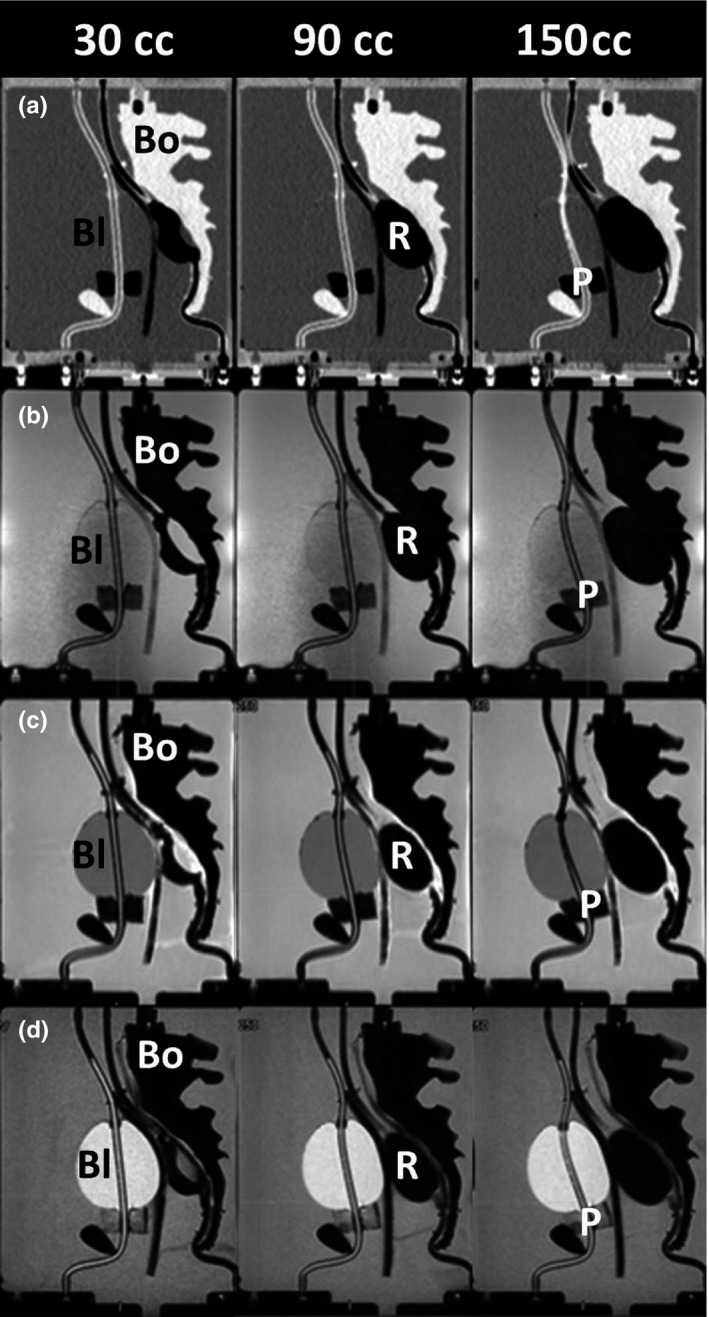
Increasing rectal volumes: sagittal view for increasing rectum volumes (30, 90, and 150 mL) for (a) CT‐SIM, (b) MR‐Linac, (c) T1W MR‐SIM, and (d) T2W MR‐SIM. The bladder (Bl), rectum (R), prostate (P), and bone (Bo) are labeled in each modality.
